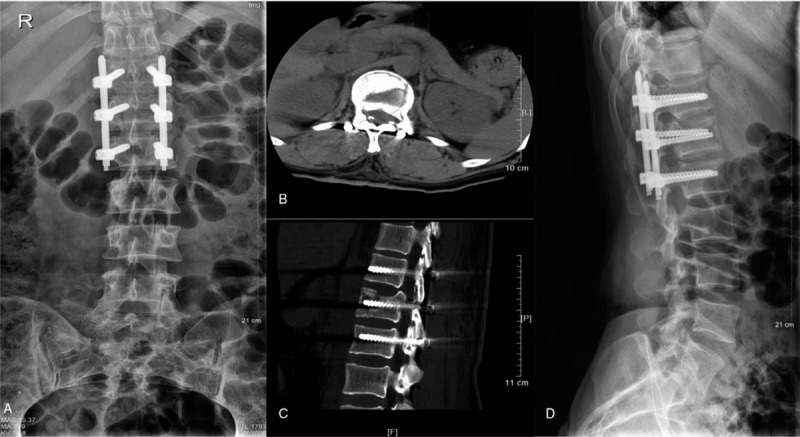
The images of the patient were re-examined on the first day after PPSF. The first postoperative radiograph showed satisfactory position of the internal fixation system (A and D). The axial (B) and sagittal (C) of the computed tomography showed the size of fracture fragments in the spinal canal decreased.
